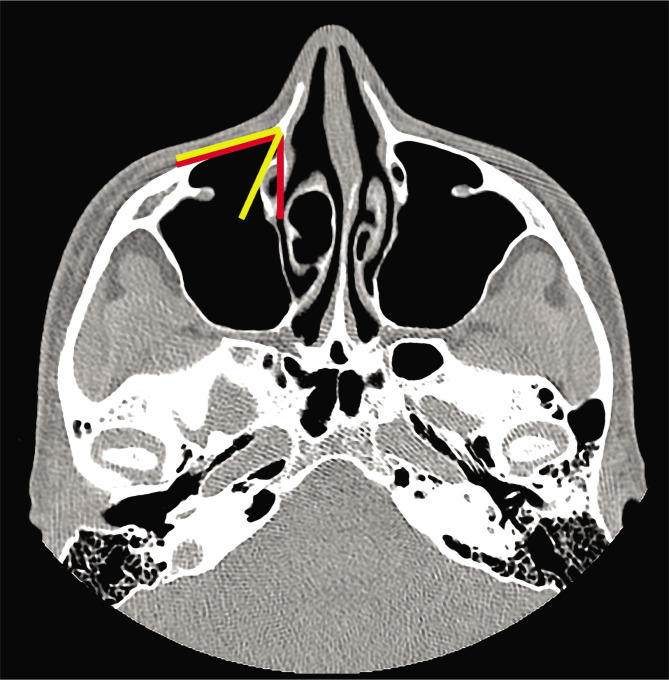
Transverse plane CT scan showing the measurements of the angles: the angle between the anterior and medial maxillary walls (angle 1) – red lines, and the angle between the anterior maxillary wall and the lateral margin of the nasolacrimal duct (angle 2) – yellow lines.
